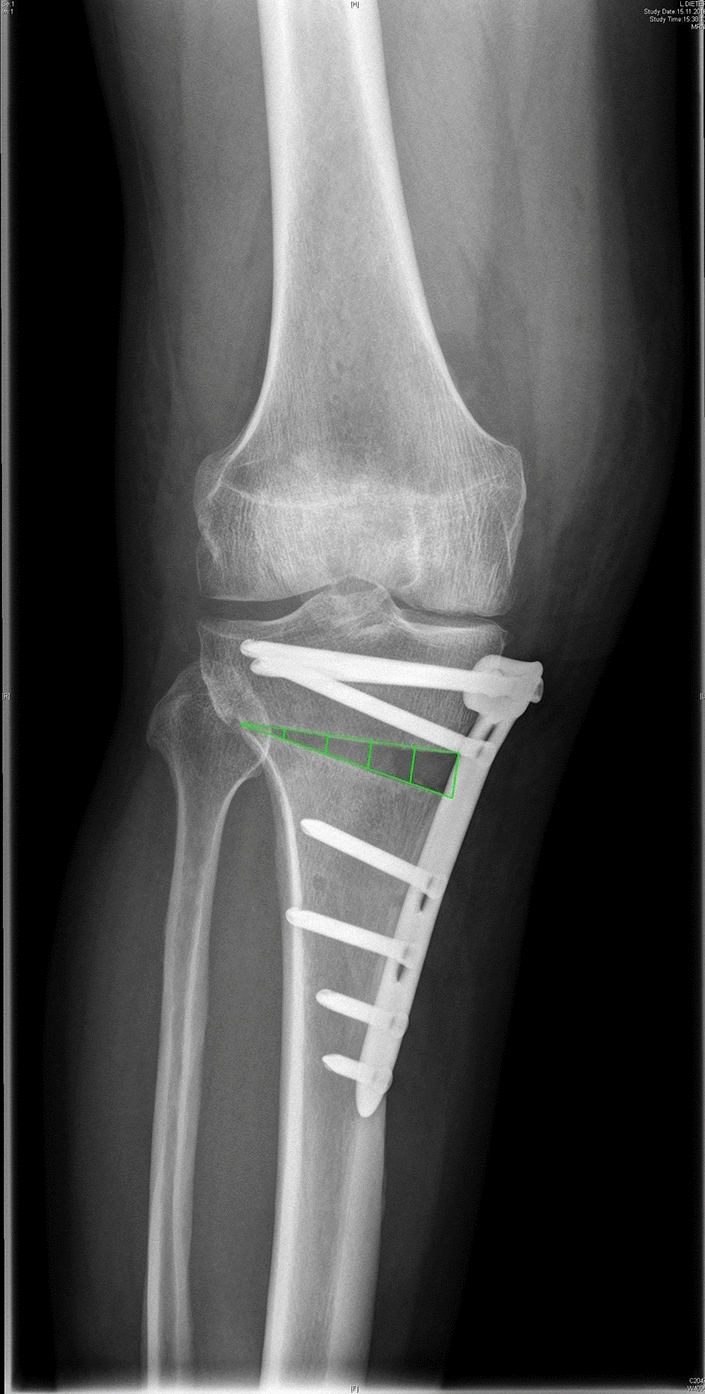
Radiographic Evaluation of an anteroposterior x-ray of a patients’ knee three days postoperatively. A triangle form of the osteotomy gap was divided into fifths by vertical lines, counting from the lateral to the medial cortex, to display the progress of consolidation. A fifth was rated as successfully consolidated when more than 50% of it was filled with bone
